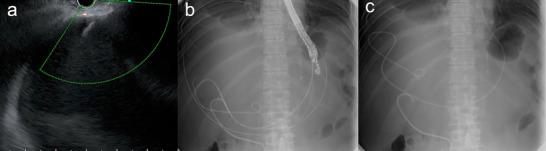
Transgastric endoscopic ultrasound‐guided cyst drainage for hepatic cyst. (a) The hepatic cyst was directly punctured from the gastric lumen under endoscopic ultrasound guidance. (b) After adequate placement of the guidewire within the giant hepatic cyst, an endoscopic nasobiliary drainage tube was successfully inserted. (c) An endoscopic nasobiliary drainage tube adequately placed within the hepatic cyst and a transpapillary biliary stent.
